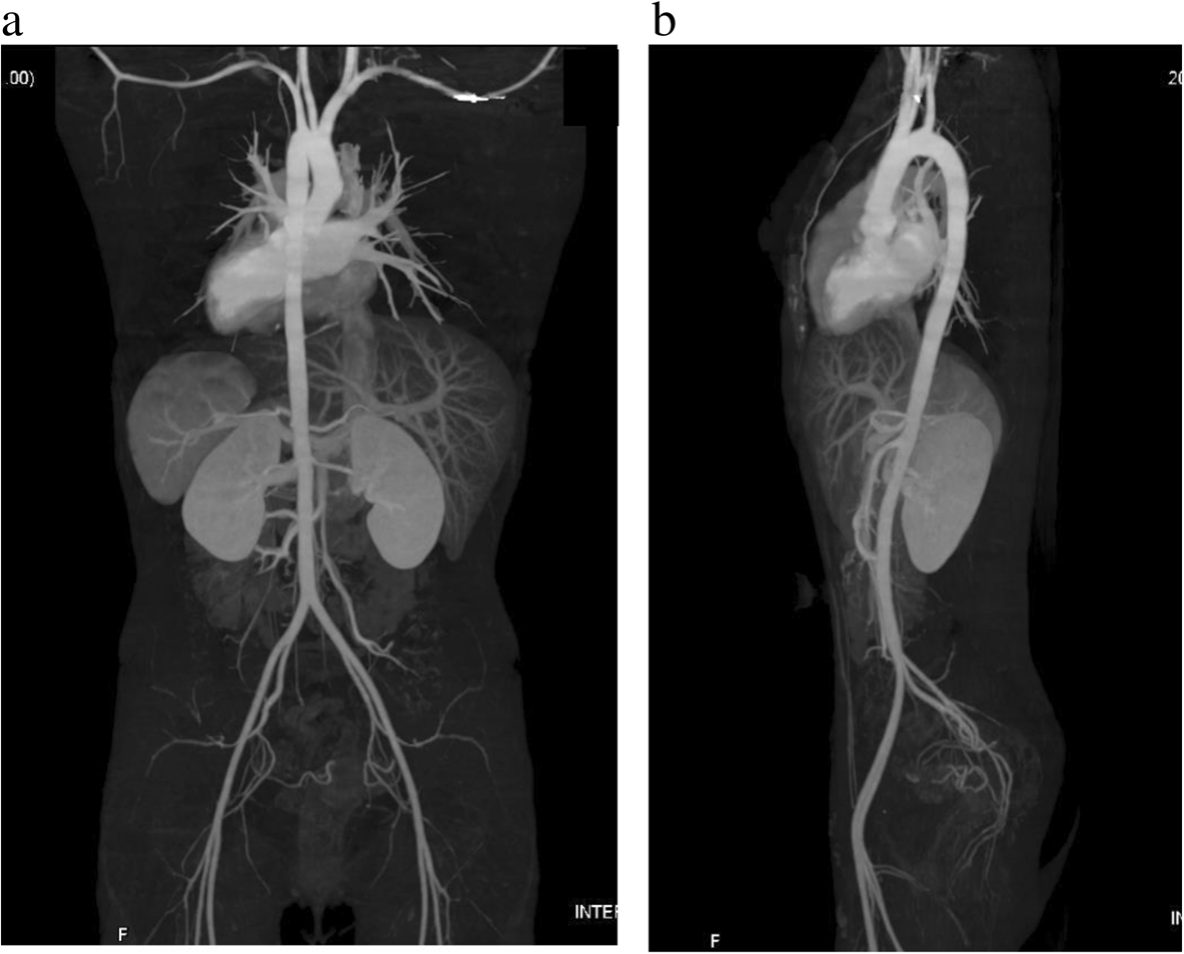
Contrast-enhanced computed tomography. a, b. No significant aortic lesion was detected by contrast-enhanced computed tomography
